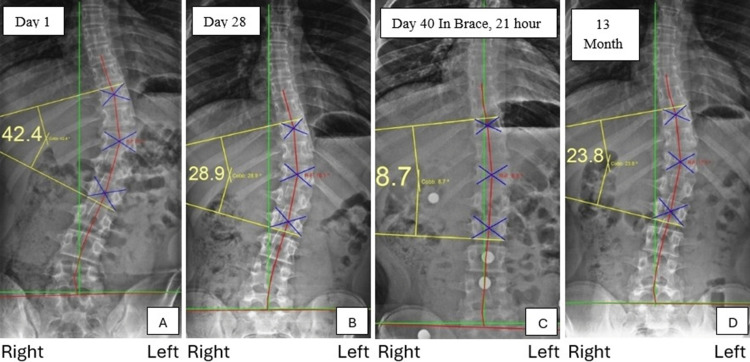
AP scoliosis radiographs Anterior-posterior (AP) full spine radiographs. Panel A is before treatment. Panel B is after treatment. Panel C is the 40-day brace radiograph. Panel D is the 13-month follow-up. The yellow lines are the Cobb measurement of scoliosis. The green lines are the ideal balance of the ideal spine. The red lines are the Risser-Ferguson analysis of the patient’s spine. The results show improvements in the Cobb angle and improved spinal coronal balance. All measurements were examined with PostureRay® software.
